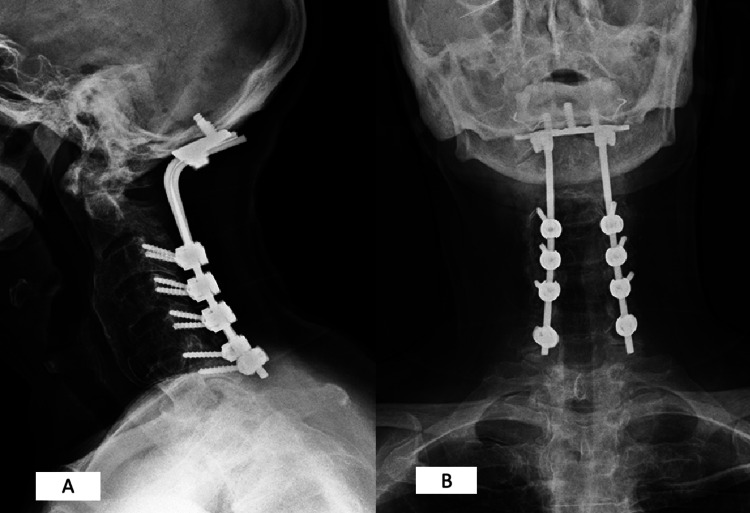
Post-operative radiography. C1-C6 laminectomy and occipito-C6 posterior fixation. (A) Lateral radiography. (B) Anteroposterior radiography
